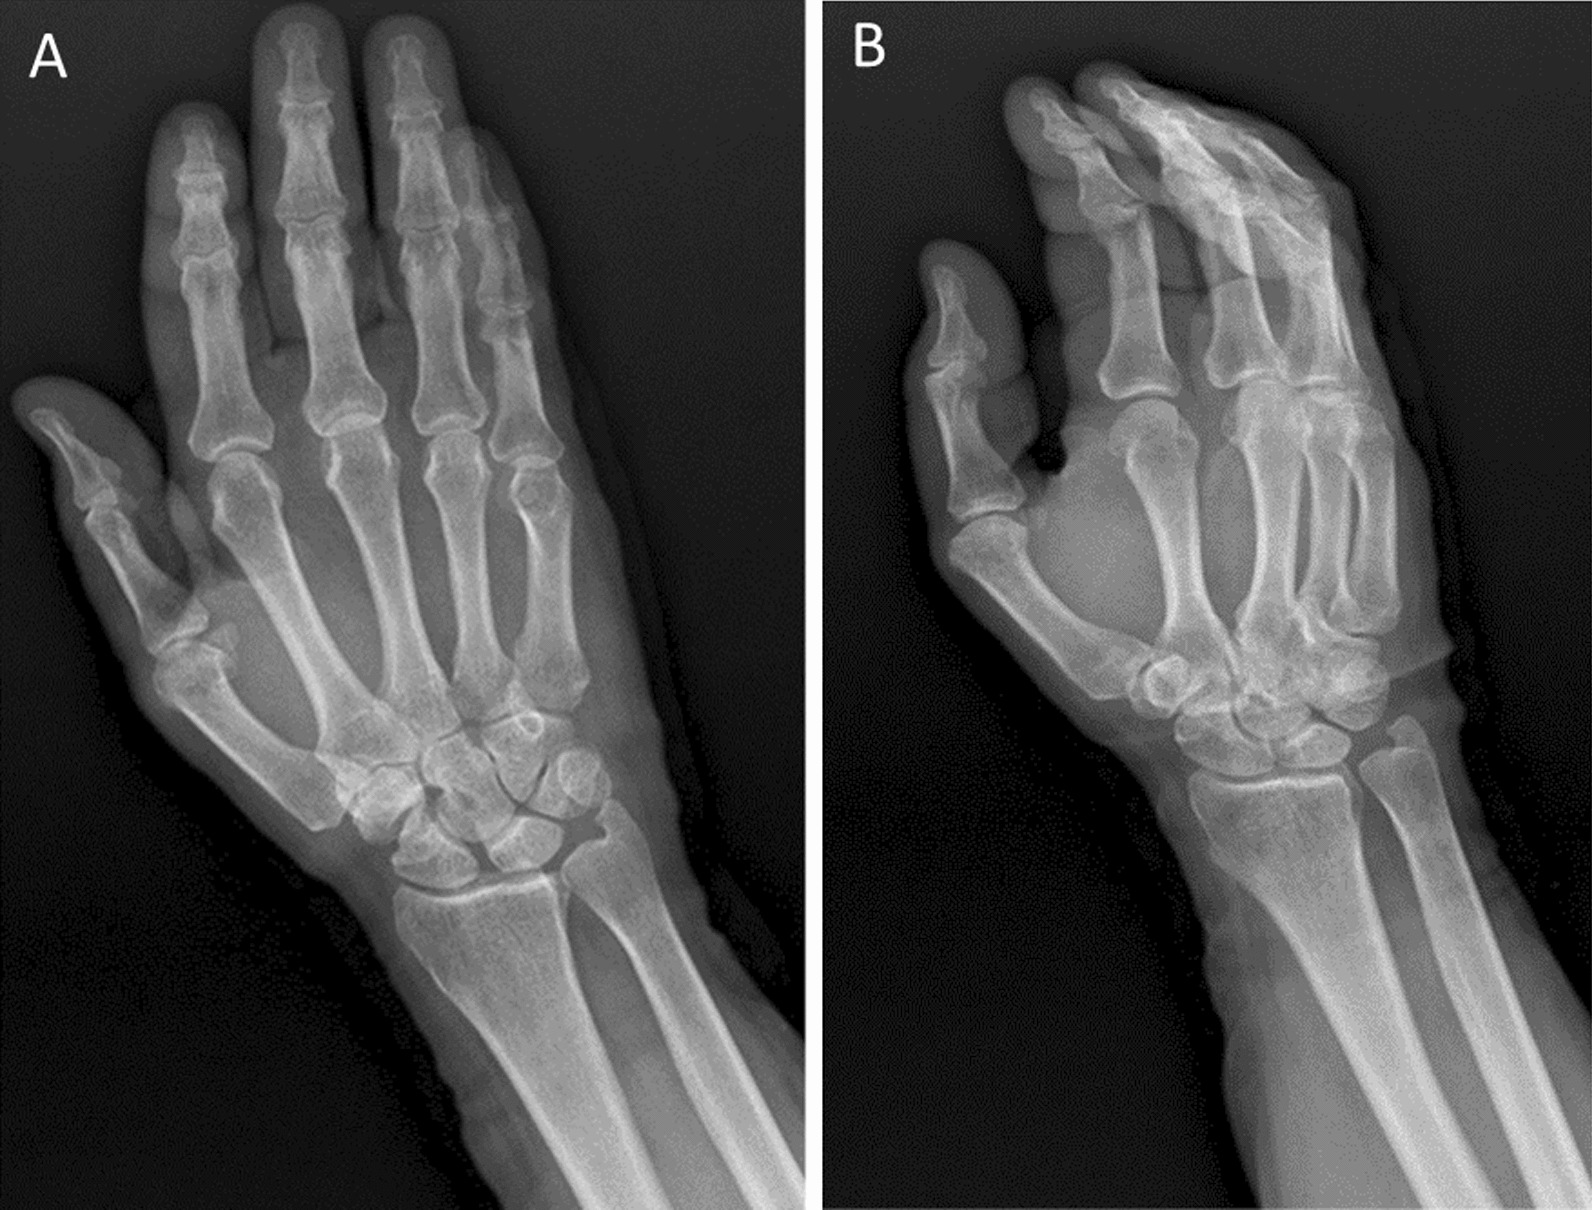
Postoperative imaging after glass removal. A Posterior-anterior hand radiograph showing interval removal of the calcified lesion. B Oblique perspective demonstrating postsurgical changes at the fifth metacarpophalangeal joint space after glass foreign body removal
